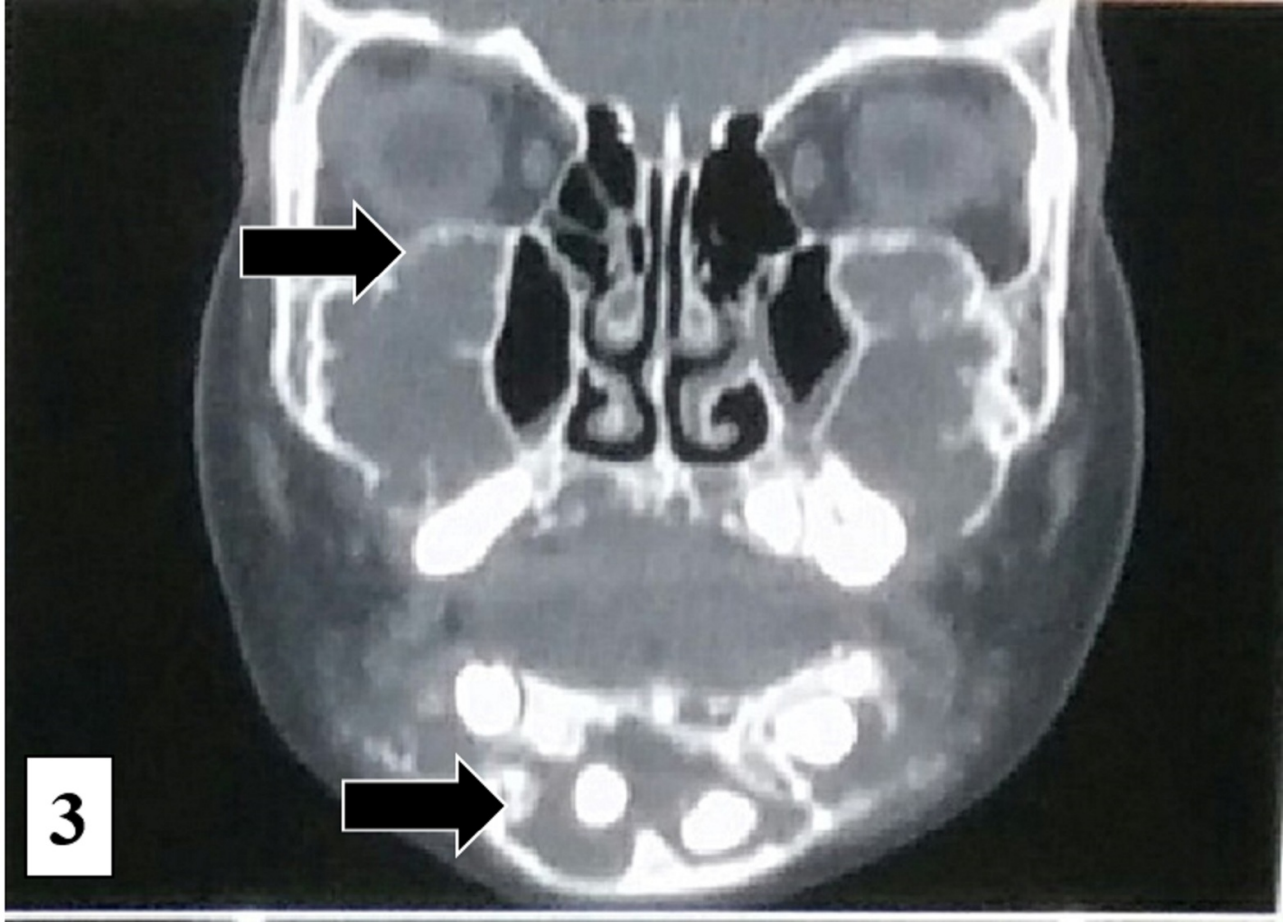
Computed Tomography in the Coronal Section A large, extensive, lytic lesion with soft-tissue density involving the entire mandible, maxilla, and lifting of the orbital floor.
